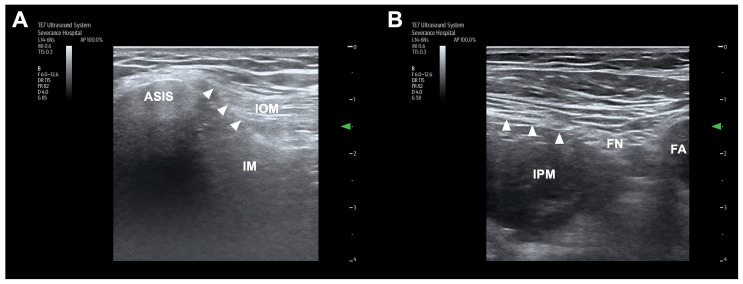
Ultrasound anatomy for the suprainguinal (A) and infrainguinal (B) fascia iliaca block. Arrowheads indicate the fascia iliaca. ASIS, anterior superior iliac spine; IM, iliacus muscle; IOM, internal abdominal oblique muscle; IPM, iliopsoas muscle; FN, femoral nerve; FA, femoral artery.
